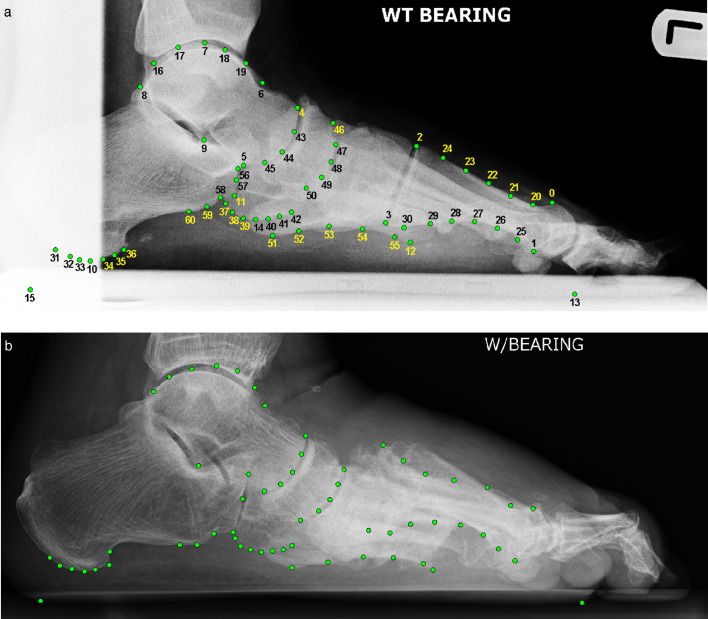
Annotation examples with 61 points (manually placed): (a) showing the 61 point positions and point indices for a *mild* case based on Meary's angle (MMA: 11.0°) with poor collimation. (b) showing the 61 point positions for a *mild* case based on Meary's angle (MMA: 6.3°) which shows advanced midfoot collapse. In this case, the 1st metatarsal has subluxed superiorly causing Meary's angle to be almost normal. This is a *severe* case based on clinical review. All radiographs have been cropped for better visualisation (MMA = mean Meary's angle over all five clinical experts).
